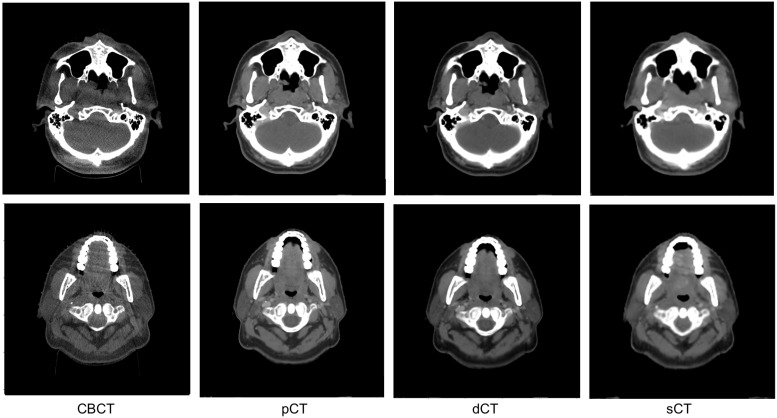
Qualitative comparison of CBCT, dCT, pCT and sCT from two different axial slices in the test set. sCT was generated from the best performing model (UNIT with RegNet).
